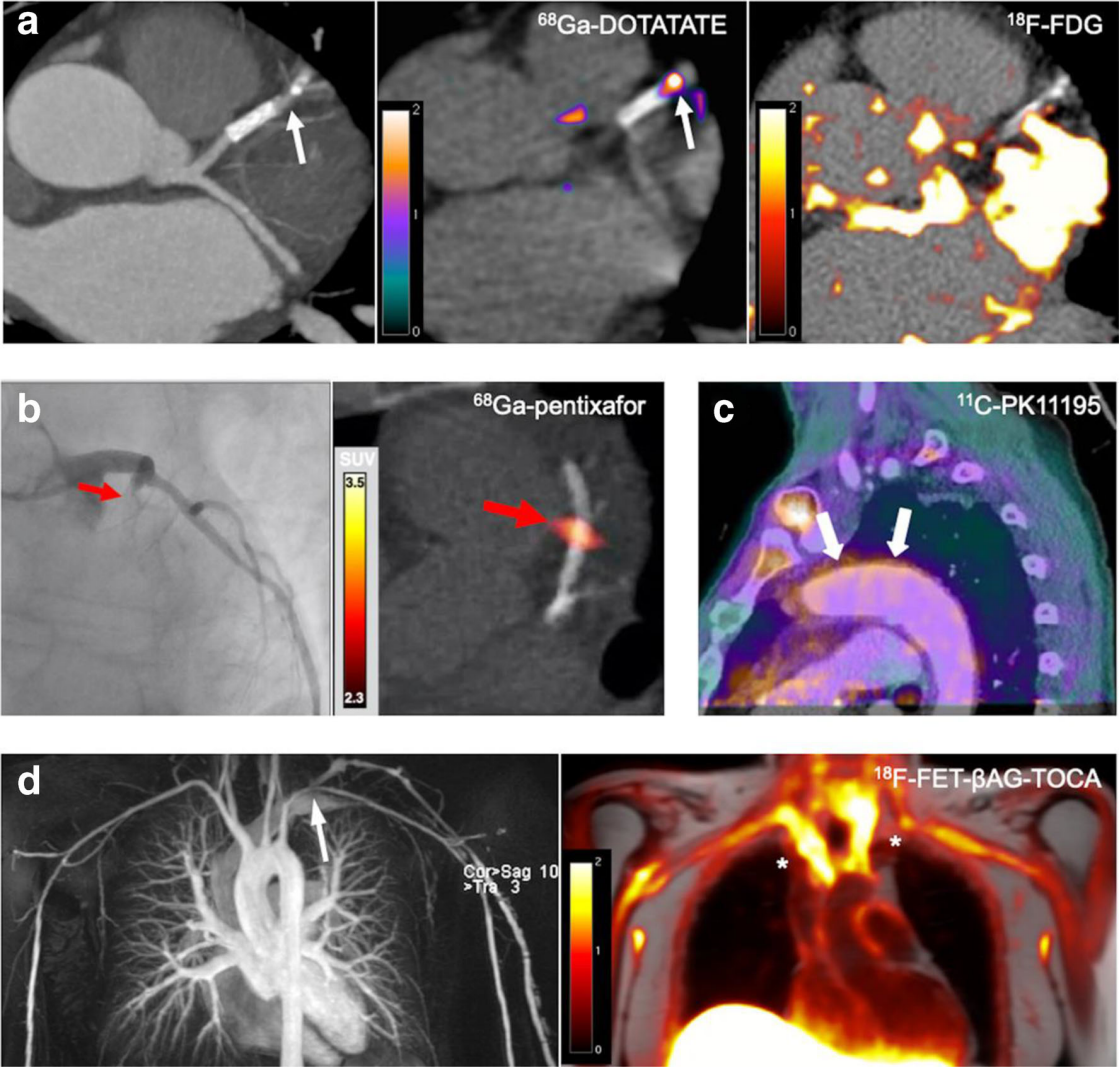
PET imaging of vascular inflammation in atherosclerosis and large-vessel vasculitis. a CT coronary angiography (left panel), ^68^Ga-DOTATATE (centre panel), and ^18^F-FDG (right panel). PET/CT imaging in a patient with non-ST segment myocardial infarction due to a culprit left anterior descending artery lesion (arrow). While there is increased ^68^Ga-DOTATATE (SST_2_) PET signal arising from the culprit coronary artery, accurate coronary ^18^F-FDG image interpretation is precluded by diffuse background myocardial tracer uptake. (Reproduced from: Tarkin JM, et al. J Am Coll Cardiol 2017; 69:1774–1791; doi: 10.1016/j.jacc.2017.01.060; Creative Commons user licence https://creativecommons.org/licenses/by/4.0/) [19•]. b Coronary angiography (left panel) and ^68^Ga-pentixafor (CXCR4) PET/CT imaging (right panel) showing avid tracer uptake in a culprit left anterior descending artery lesion (arrow) of a patient with acute myocardial infarction. (Reproduced from: Derlin T, et al. J Nucl Med Mol Img 2018; 45:1934–1944; doi.org/10.1007/s00259-018-4076-2; Creative Commons user licence https://creativecommons.org/licenses/by/4.0/) [25•]. c
